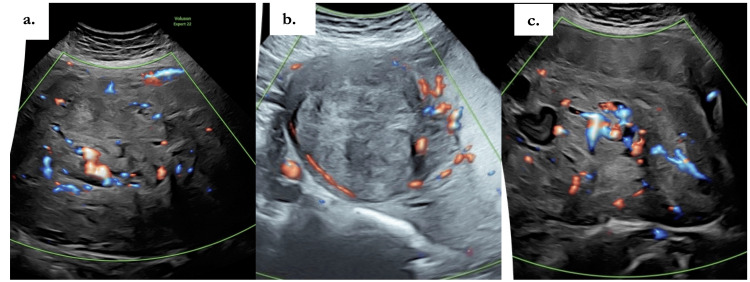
Color Doppler ultrasound in the third trimester. a: overview of a large, heterogeneous uterine mass displacing the uterus; b: peripheral vascularity along the lesion margin; c: abundant central intratumoral vascularity concerning for sarcoma.
